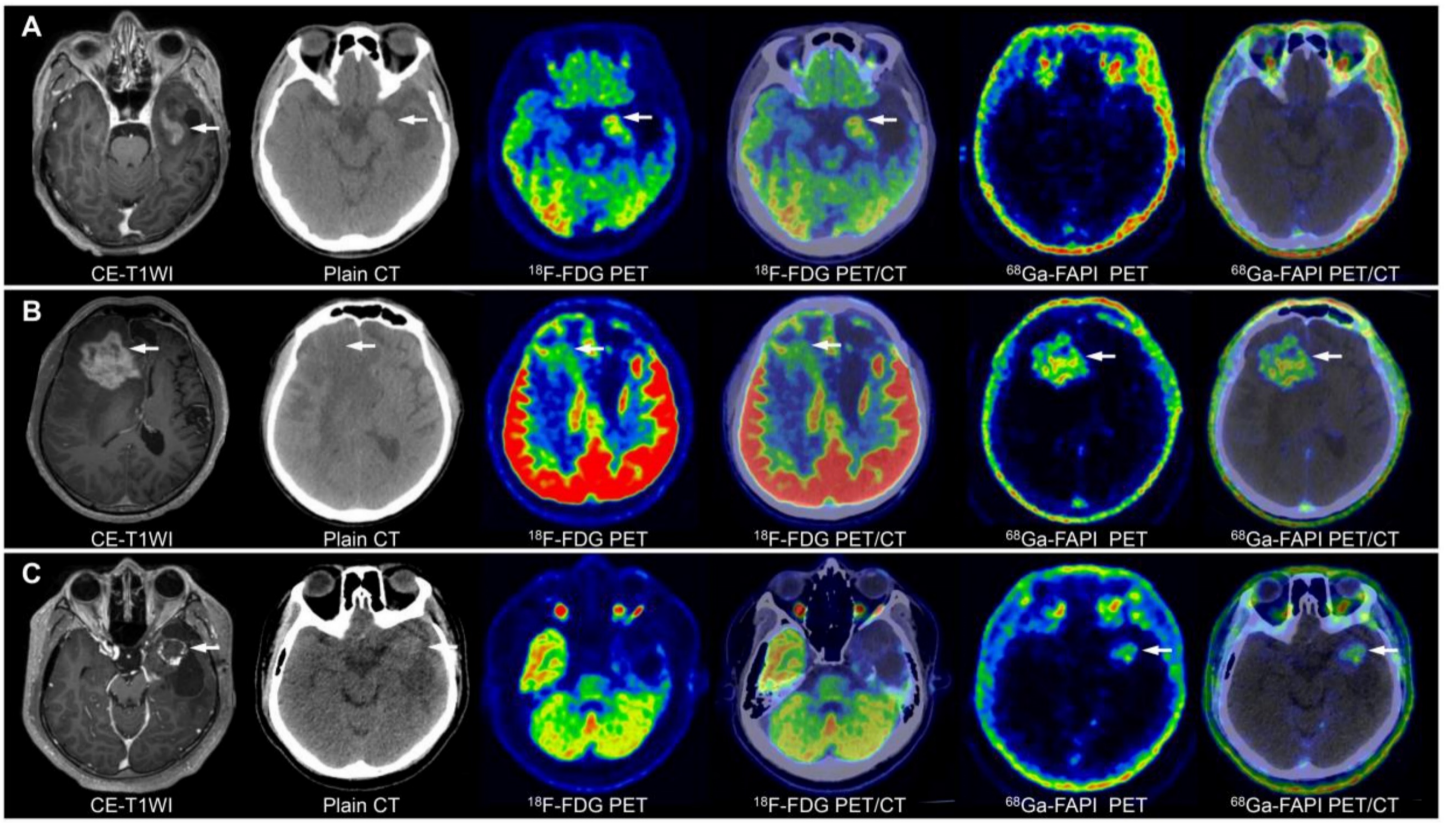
PET/CT and CE-MRI for the detection of glioma recurrence (lesions are indicated by arrows). A 44-year-old male with a history of surgery and radiotherapy for anaplastic astrocytoma had a suspicious recurrent lesion in the left temporal lobe on CE-MRI, with a significantly increased ^18^F-FDG uptake but negative ^68^Ga-FAPI-46 uptake. The follow-up MRI revealed loss of enhancement of this lesion, which confirmed no evidence of tumour recurrence (A). Another 44-year-old male with recurrent anaplastic oligodendroglioma in the right frontal lobe after surgery showed mildly increased ^18^F-FDG uptake and significantly increased ^68^Ga-FAPI-46 uptake, with the lesion visible on ^68^Ga-FAPI-46 PET/CT (B). A 30-year-old male with recurrent glioblastoma in the temporal lobe after surgery exhibited contrast-enhanced foci on CE-T1WI in the surgical area, with mild tracer uptake on ^18^F-FDG PET/CT and significantly increased tracer uptake on ^68^Ga-FAPI-46 PET/CT (C).
